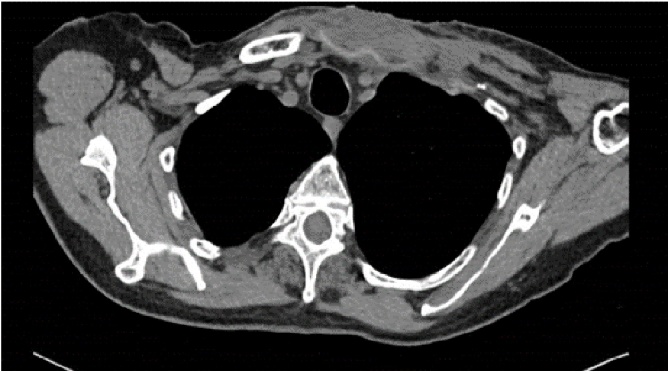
CT after the operation. There is residual, poorly defined soft tissue within the surgical bed, which is thought to represent a combination of post-surgical change and interval radiotherapy, rather than recurrent or residual tumour.
